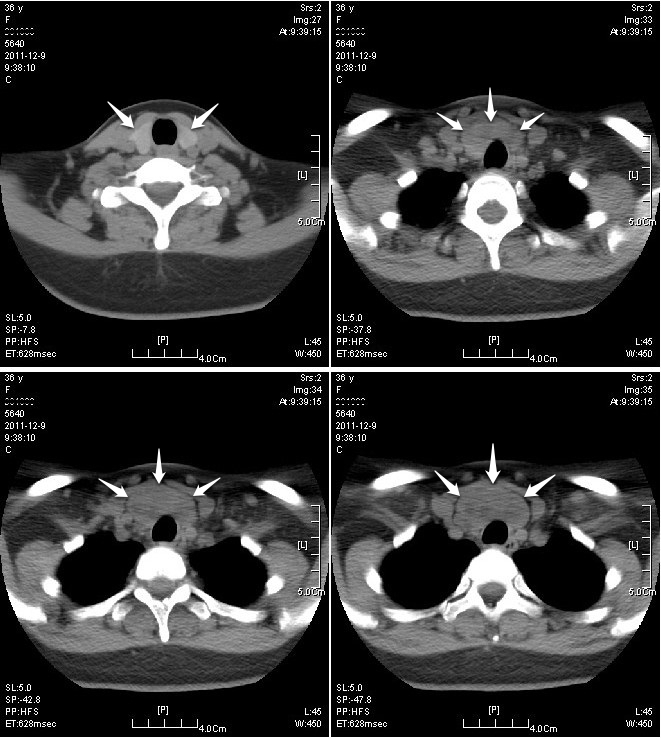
Computed tomography (CT) scans of the patient’s neck revealed a well-defined mass below the thyroid gland on the border between the cervix and the thorax.
